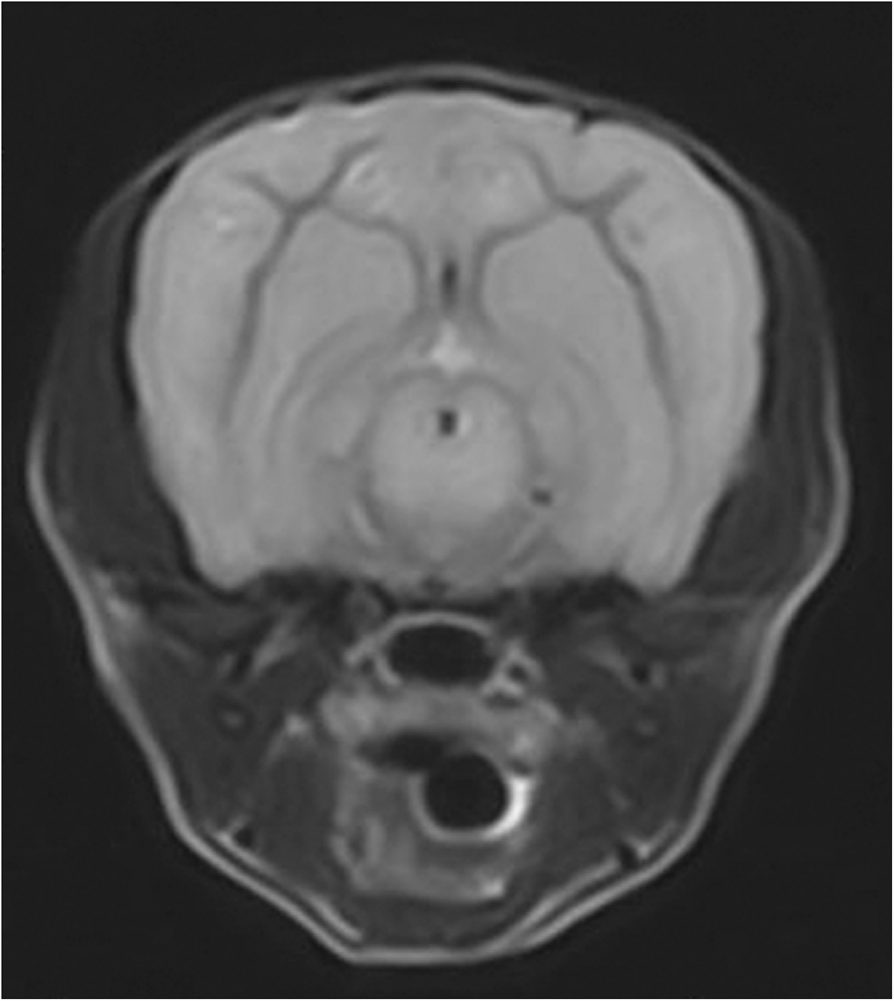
Transverse T2-weighted image (TR = 3000, TE = 90) through the midbrain of the Yorkshire terrier with L-2-HGA. The gray matter of the cerebrum and brainstem shows the symmetric hyperintensity characteristic of the disease [7,9].
